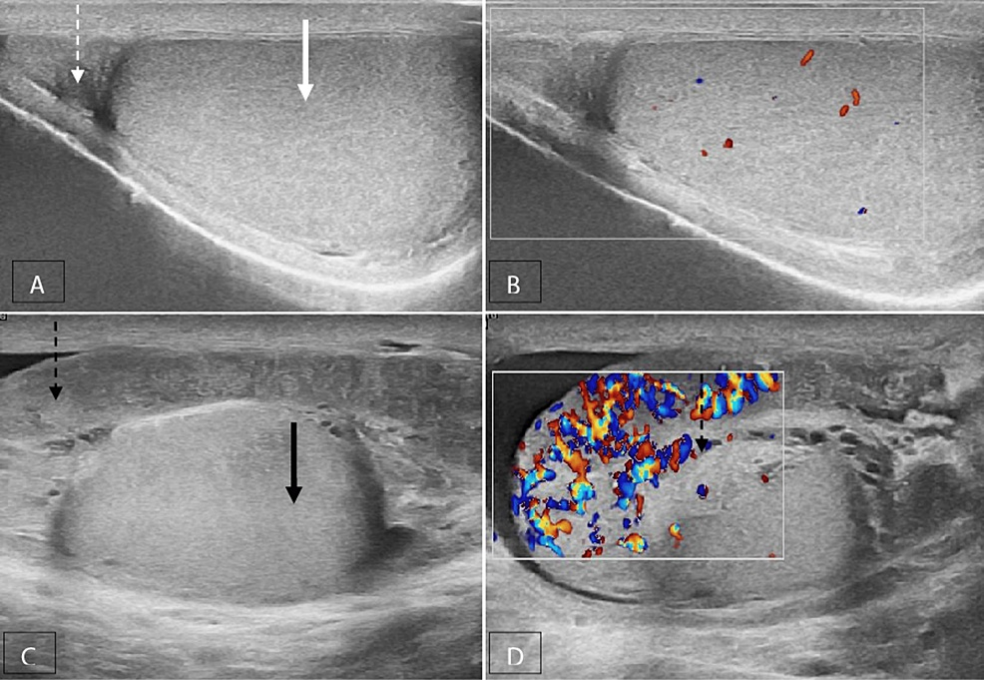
Acute epididymitis A, B Grey-scale and colour Doppler images of a normal right testis (solid white arrow) and epididymis (dotted white arrow); C, D grey-scale and colour Doppler images of the left testis (solid black arrow) and epididymis (dotted black arrow). The left testis is normal. The left epididymis is enlarged in size and shows heterogeneous echotexture with increased vascularity.
